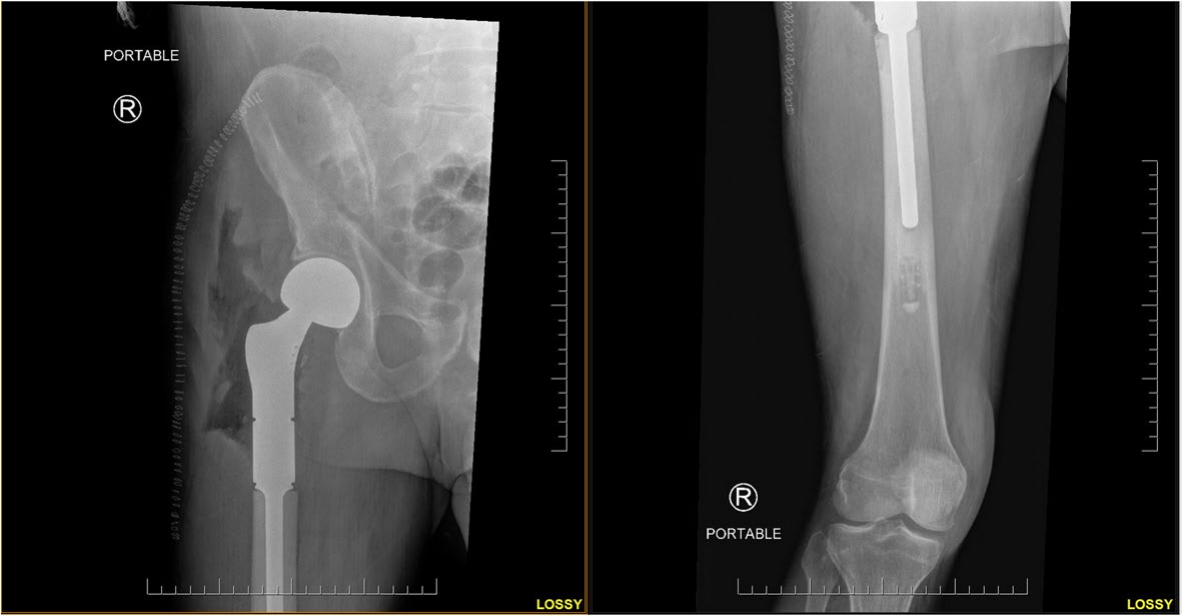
An AP radiograph showing a proximal femoral replacement using a megaprosthesis implant
